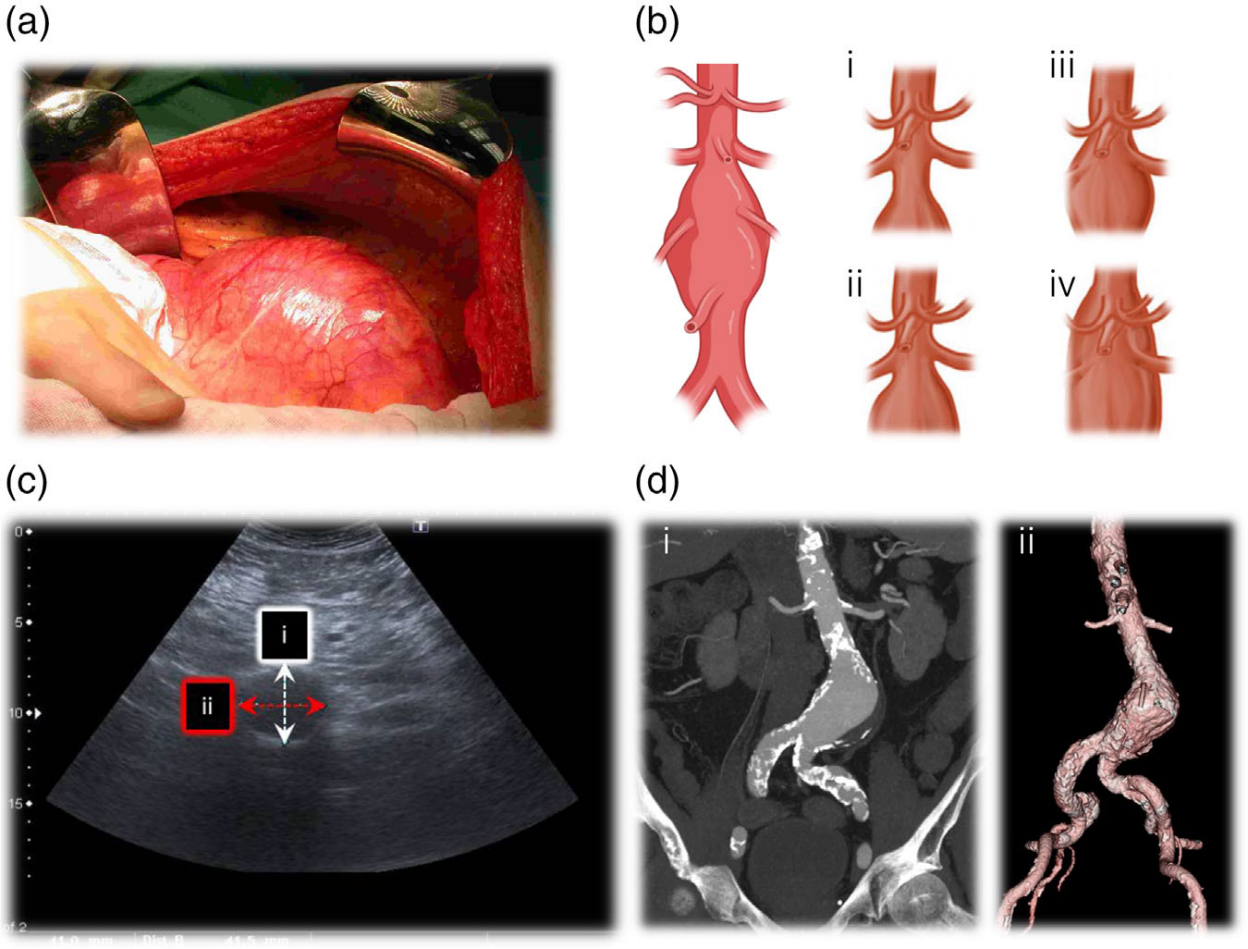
Clinical presentation of an abdominal aortic aneurysm (AAA). (a) Surgical appearance of a large (8.5‐cm‐diameter) infrarenal AAA during open transperitoneal repair. (b) Anatomical classification of AAAs: i, infrarenal AAA; ii, juxtarenal AAA; iii, suprarenal AAA; iv, type IV thoracoabdominal aortic aneurysm. (c) Appearance on ultrasound (transverse plane), enabling assessment of anteroposterior (i) and transverse (ii) diameters. (d) Coronal maximum‐intensity projection (i) and three‐dimensional reformat (ii) of an AAA (5.5 cm in diameter). Note the adequate length of the iliac arteries and the long infrarenal neck below the renal arteries, highlighting suitability for endovascular repair. (d) Adapted from (Swerdlow et al., 2019).
